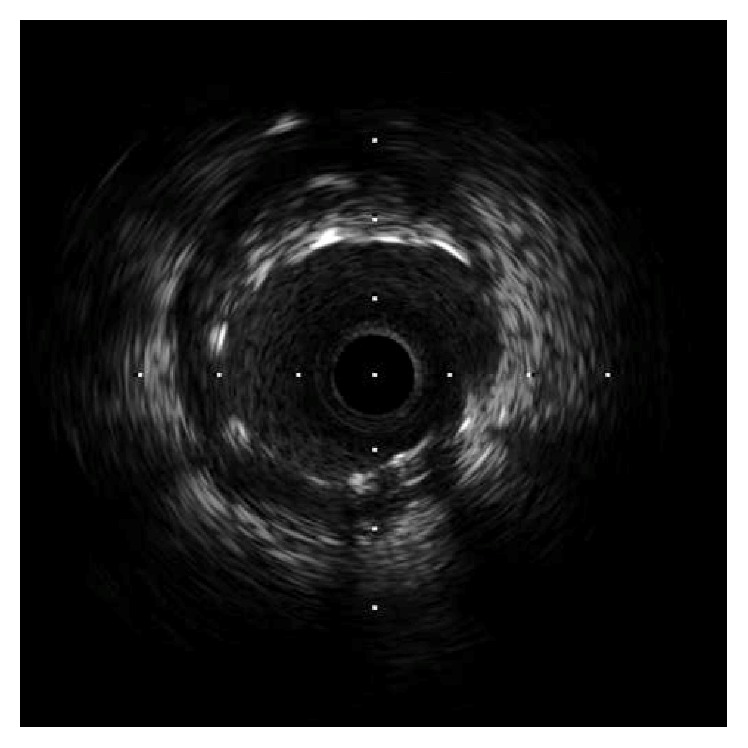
Repeat intravascular ultrasound imaging revealed improved apposition of the stent strut.
